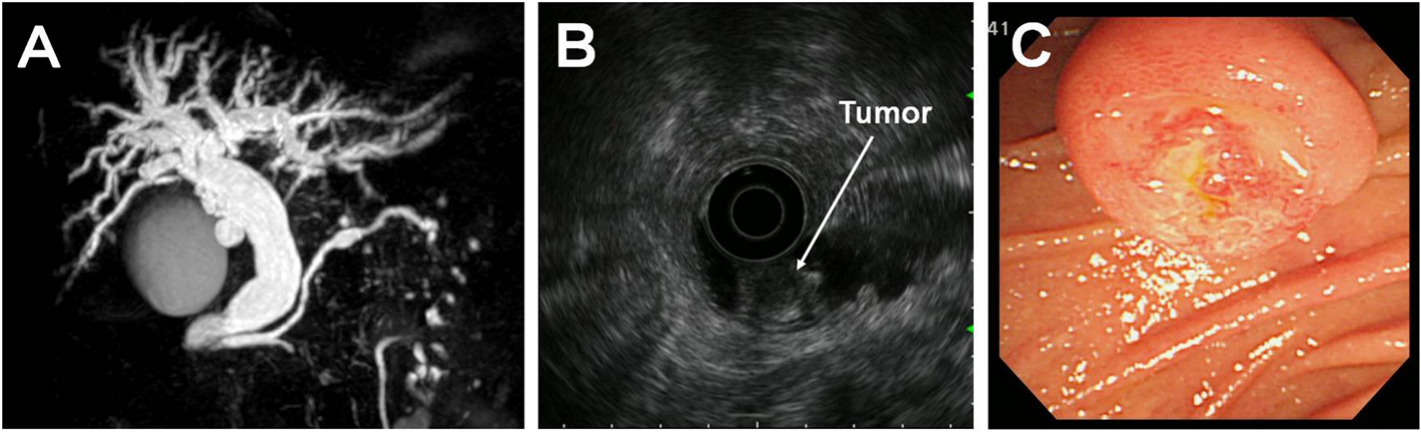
a Magnetic resonance cholangiopancreatography showed dilatation of the intra- and extrahepatic bile ducts and the main pancreatic duct without any stricture. b Endoscopic ultrasonography showed a hypoechoic mass in the ampulla of Vater located on the inside of the duodenal wall without invasion of the duodenal muscularis propria. c Duodenoscopy revealed an exposed reddish tumor at the ampulla of Vater
